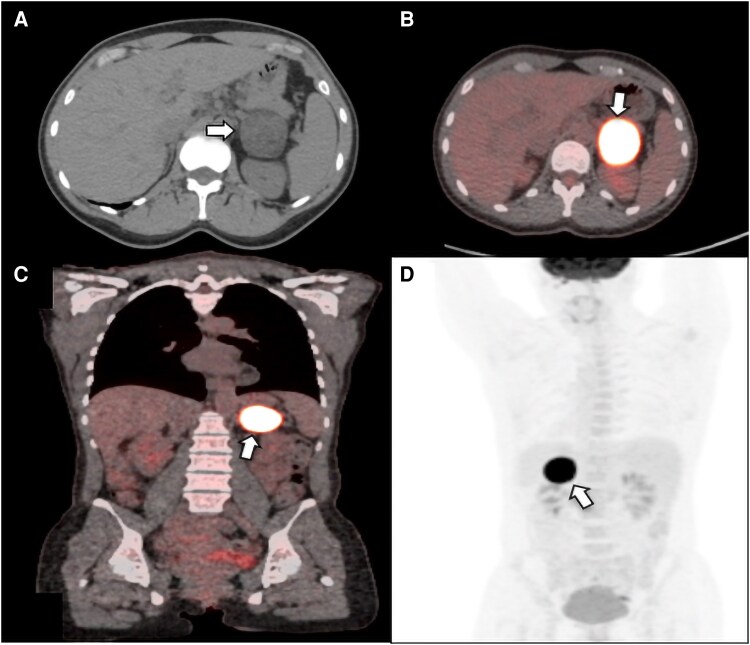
Axial computed tomography of the abdomen (A) of the same patient before intravenous contrast administration shows a 4.3 cm heterogeneous left adrenal mass (white arrow) with an unenhanced attenuation value of around 15 HU. On ^18^FDG-PET images (B-D), the lesion demonstrates avid ^18^FDG uptake with a with SUV maximum 38.7. Abbreviations: HU, Hounsfield units; ^18^FDG-PET, 18F-fluorodeoxyglucose positron emission tomography; SUV, standardized uptake value.
